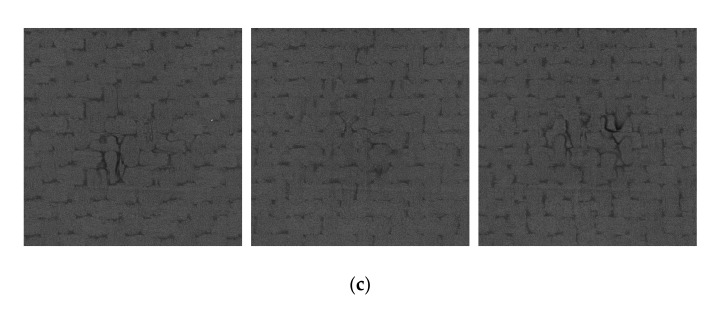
Fragments of raw non-destructive testing (NDT) results obtained for the selected damage case (E17.5): the ultrasonic C-Scan (a); selected sections of the ultrasonic B-Scan (b), and the X-ray computed tomography (CT) scan (c).
